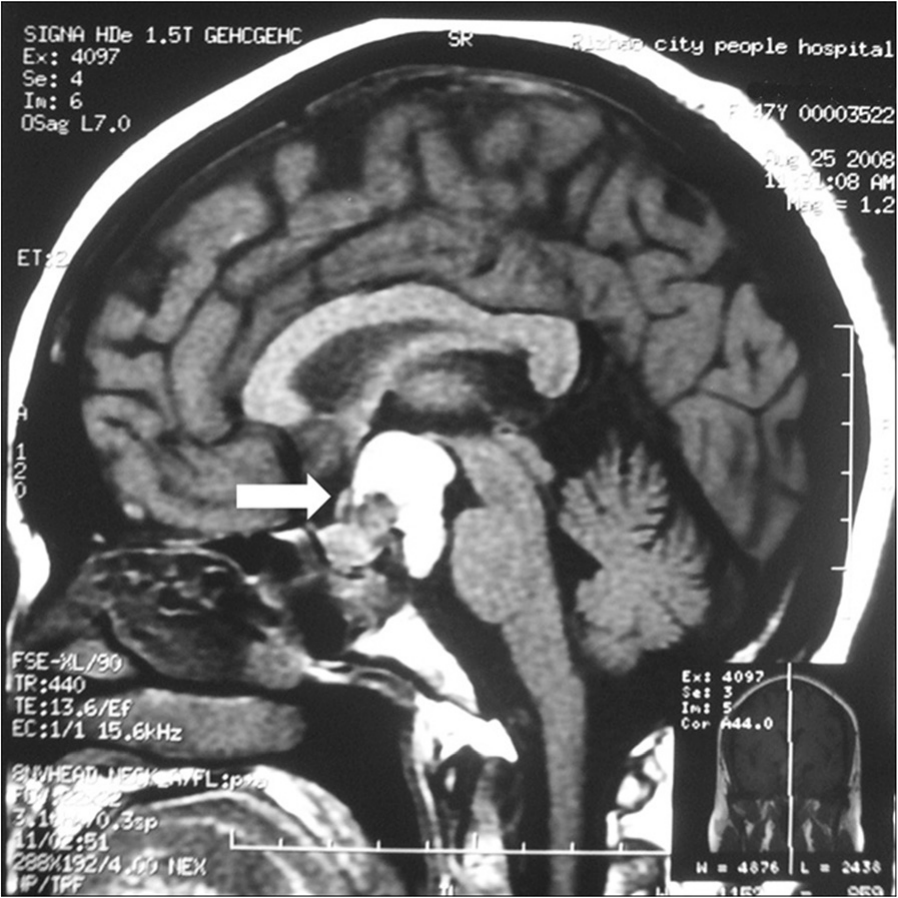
Post-operative MRI scan showing subtotal tumor resection (arrow indicated the enlarged cystic mass).
